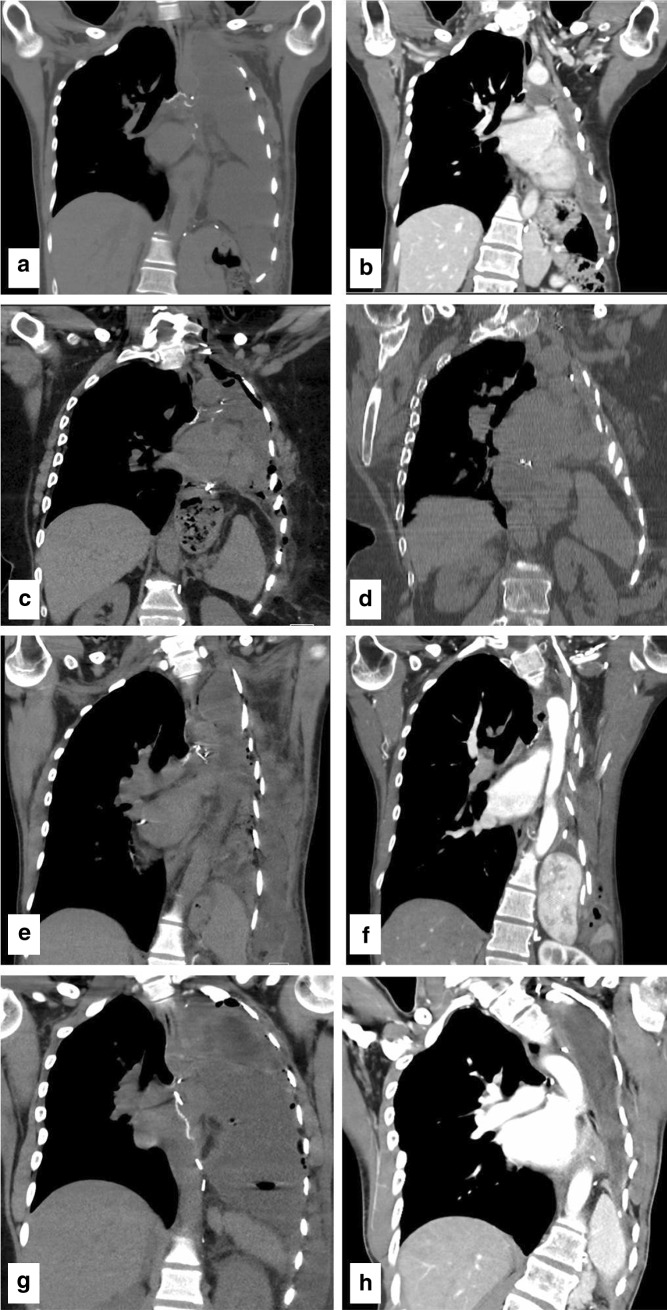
Postoperative coronal view of a chest computed tomography scan at the carinal bifurcation/left bronchial stump level. Patient 1: (a) 14th postoperative day and (b) 66 months after surgery. Patient 2: (c) 27th postoperative day and (d) 62 months after. Patient 3: (e) 9th postoperative day and (f) 60 months after. Patient 4: (g) 15th postoperative day and (h) 54 months after
